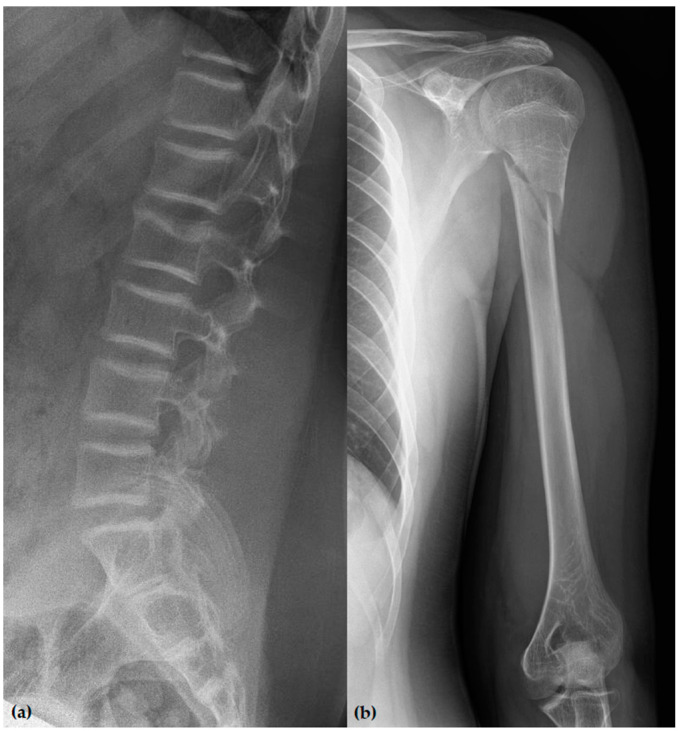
(a) Profile lumbar spine radiograph showing a compression fracture of the L2 vertebral body. The superior endplate is compressed posteriorly, with minor loss of vertebral body height corresponding to grade 1 by Genant classification. (b) Anteroposterior radiograph of the left upper arm showing a spiral fracture of the proximal third of the humerus.
